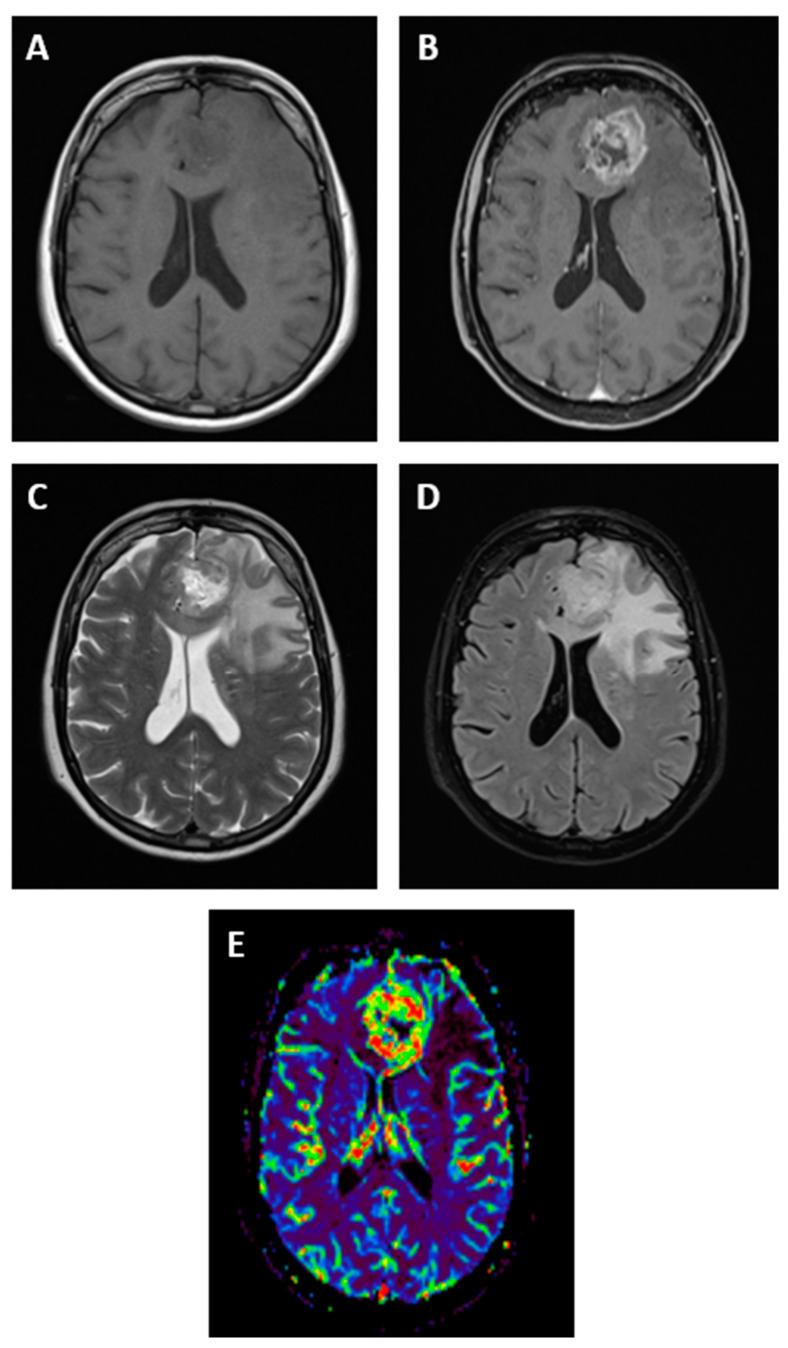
Magnetic resonance imaging features of a patient with GBM in the left frontal lobe. GBM appears as a hypointense or isointense mass on T1-weighted images (A), with a ring pattern of enhancement on gadolinium-enhanced images reflecting the increased blood–brain barrier permeability (B). GBM is typically hyperintense on both T2-weighted (C) and fluid-attenuated inversion recovery (FLAIR, D) images, surrounded by vasogenic edema. Perfusion-weighted imaging is an advanced MRI method useful in GBM for differential diagnosis (E).
